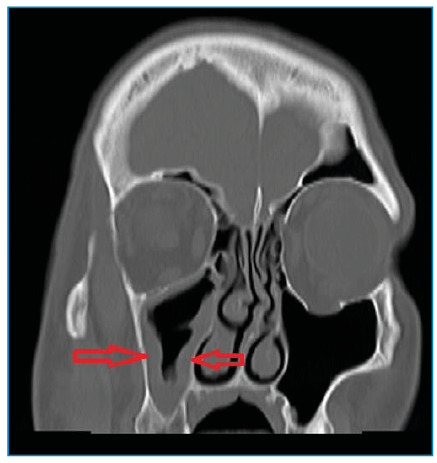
A paranasal computed tomography scan showing mucosal thickening in the right maxillary sinus.
